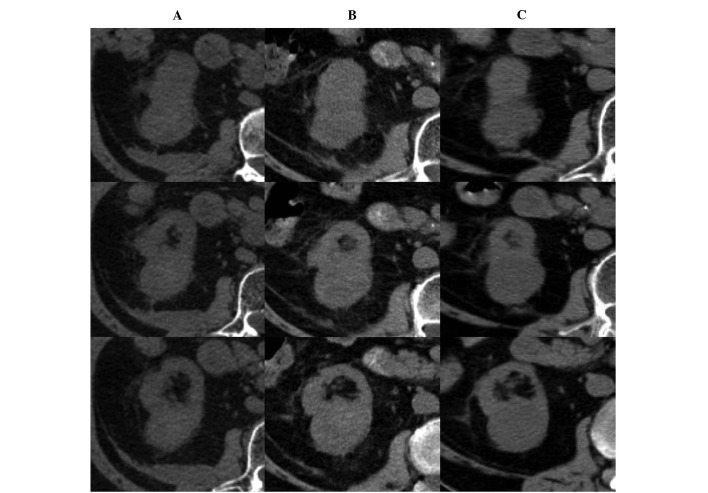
Computed tomography (CT) scan of patient 1 with renal cell carcinoma prior to and following stereotactic ablative radiotherapy (SABR). (A) Unenhanced scan prior to treatment shows a well-defined protruding mass in the right kidney, as well as a thin cortex and irregular contour of the kidney, which is consistent with chronic kidney disease. (B) CT scan 12 months and (C) 24 months following SABR. The patient was classified as stable following radiotherapy.
